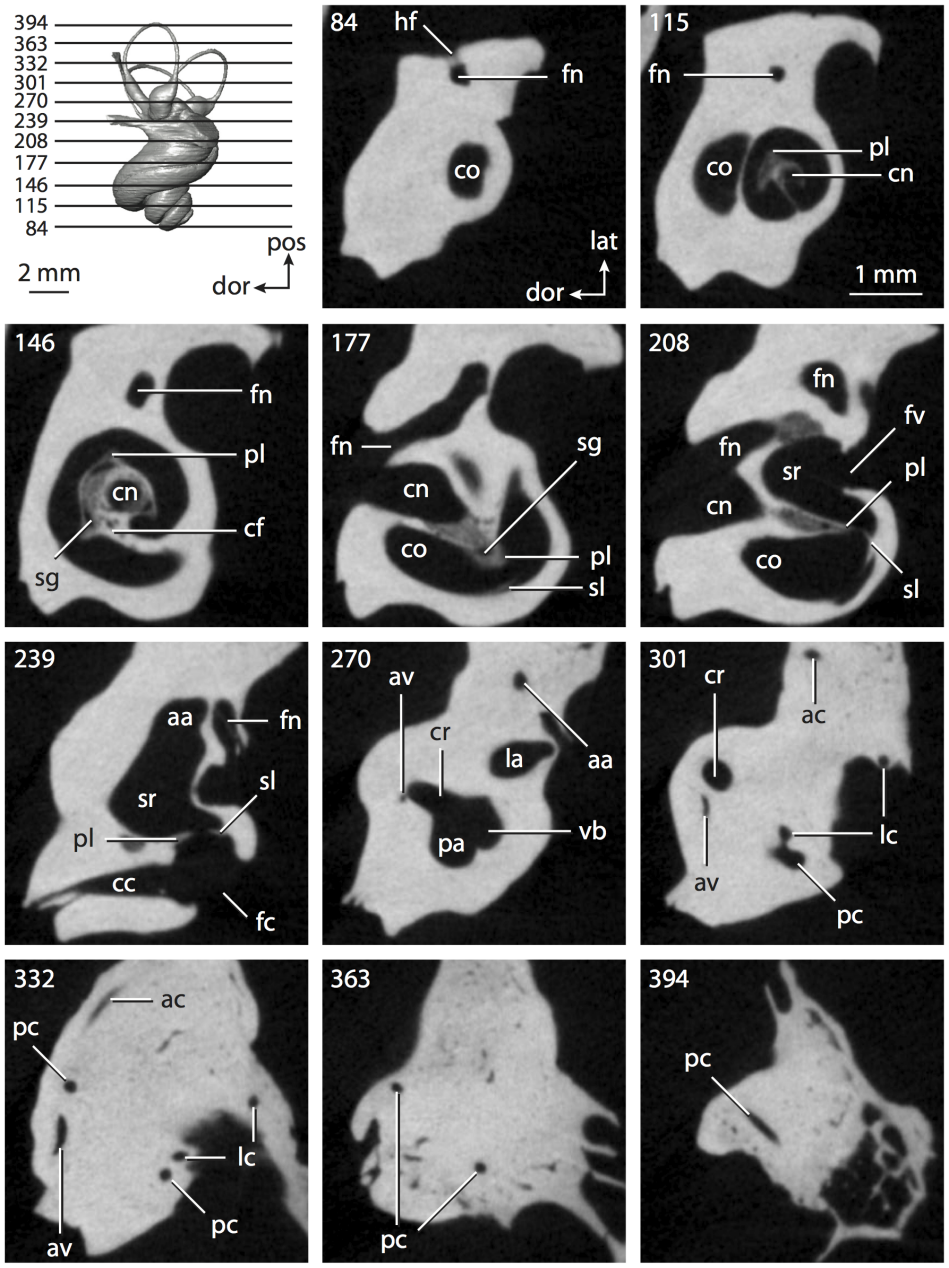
CT slices through ear region of*Felis catus*. Abbreviations listed at the end of the Materials and Methods section.
